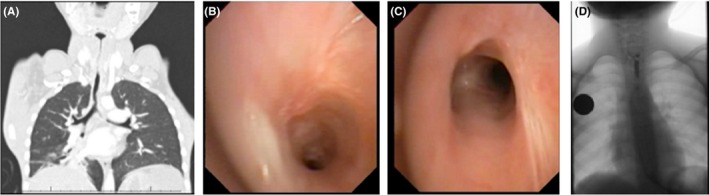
A, Coronal chest CT‐scan reconstruction: Demonstrating two levels of narrowing of the trachea, with a very narrow proximal part and irregular shape of most of the trachea. B and C, Bronchoscopy images of the proximal and distal areas of narrowing, also demonstrating severe mucosal injury. D, Contrast swallow study: Severe short segment narrowing of the esophagus
